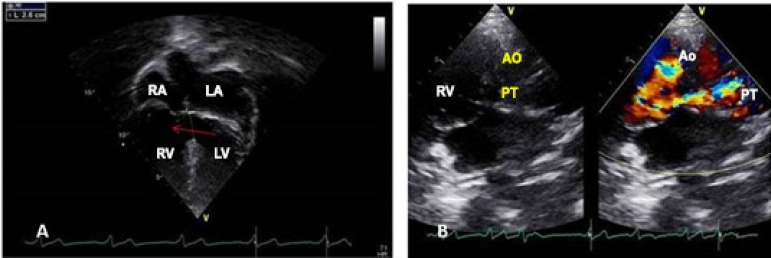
Echocardiogram shows in the 4-chamber view the large ventricular
